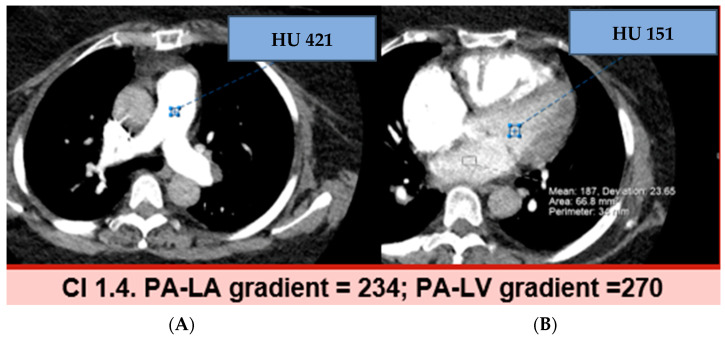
CTEPH case with low cardiac index. Post contrast CT axial image at the level of MPA with ROI in the MPA (A) and LV (B). The MPA-LV gradient was 270, which is above the cut off of 115 that correlates with low cardiac index (≥115 implies low CI).
